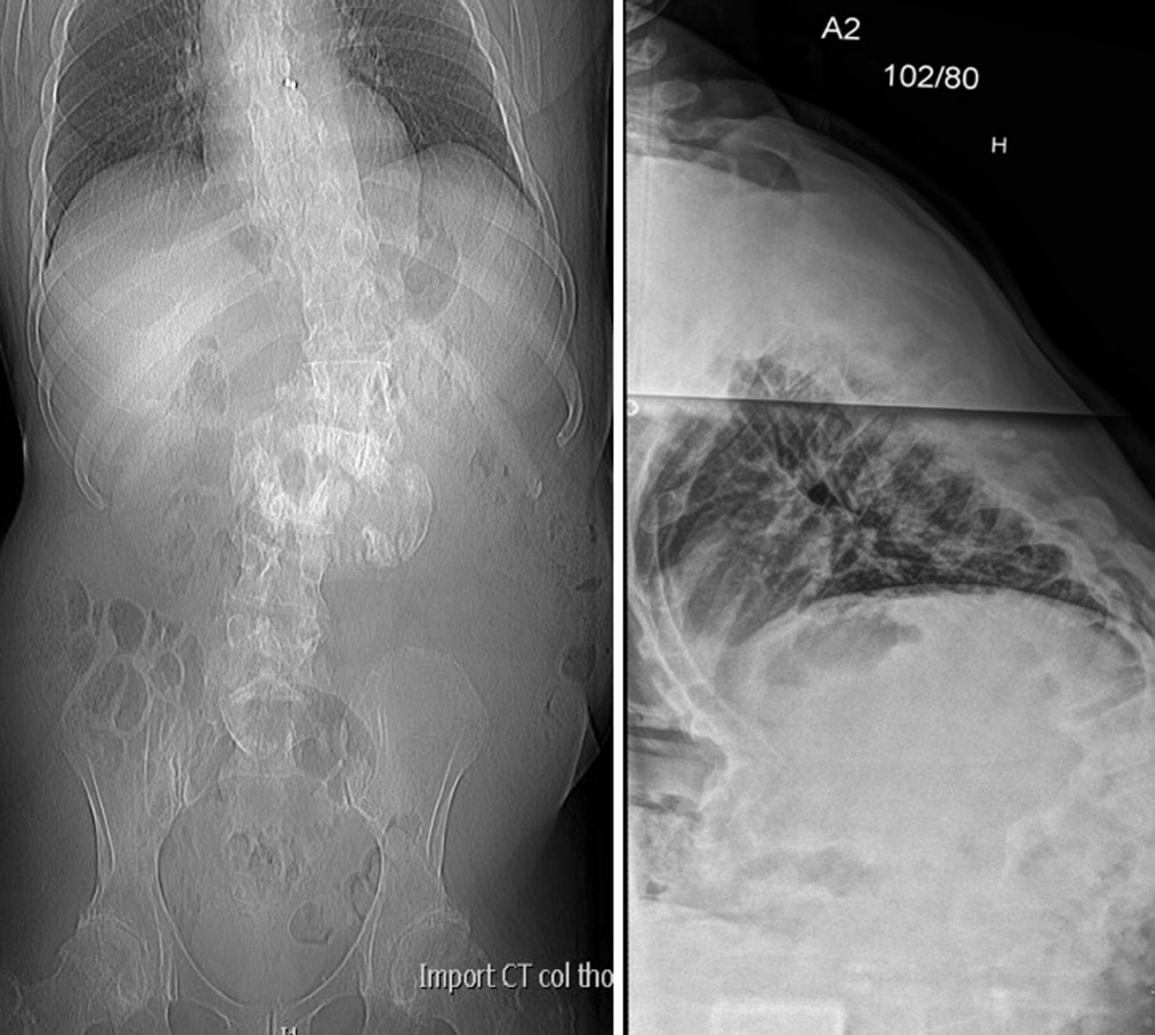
Radiographs showing marked destruction of L2 and L3 vertebrae with paravertebral hypertrophic ossification and kypho-scoliotic deformity.
